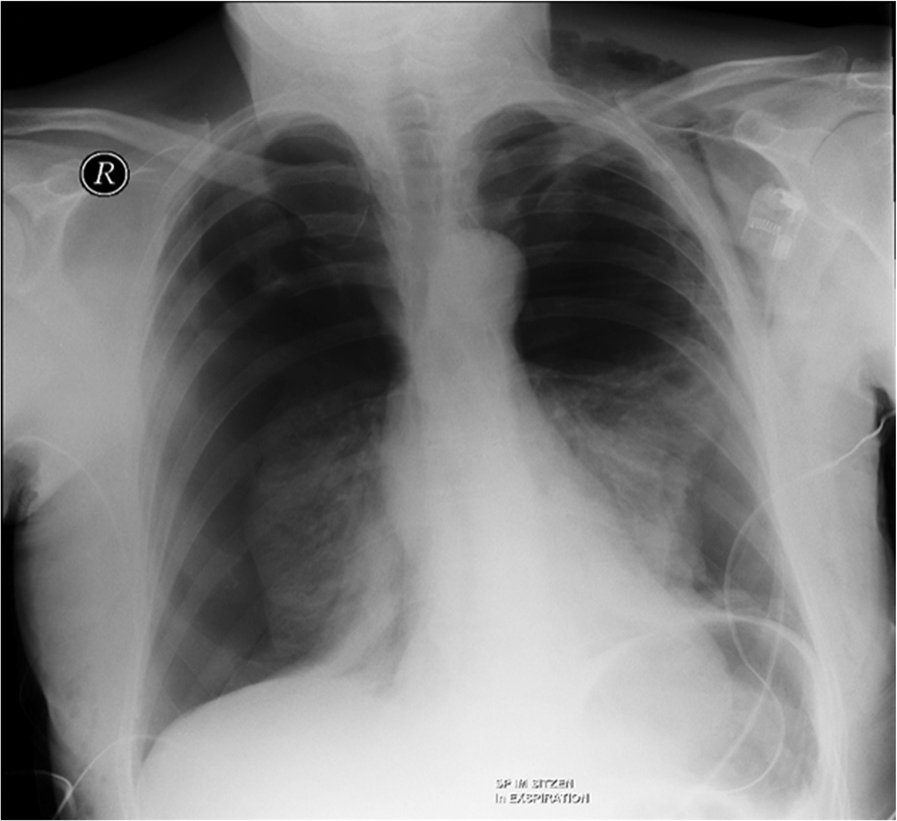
Chest radiograph at time of admission demonstrating the bilateral pneumothoraces after reduction mammoplasty.
